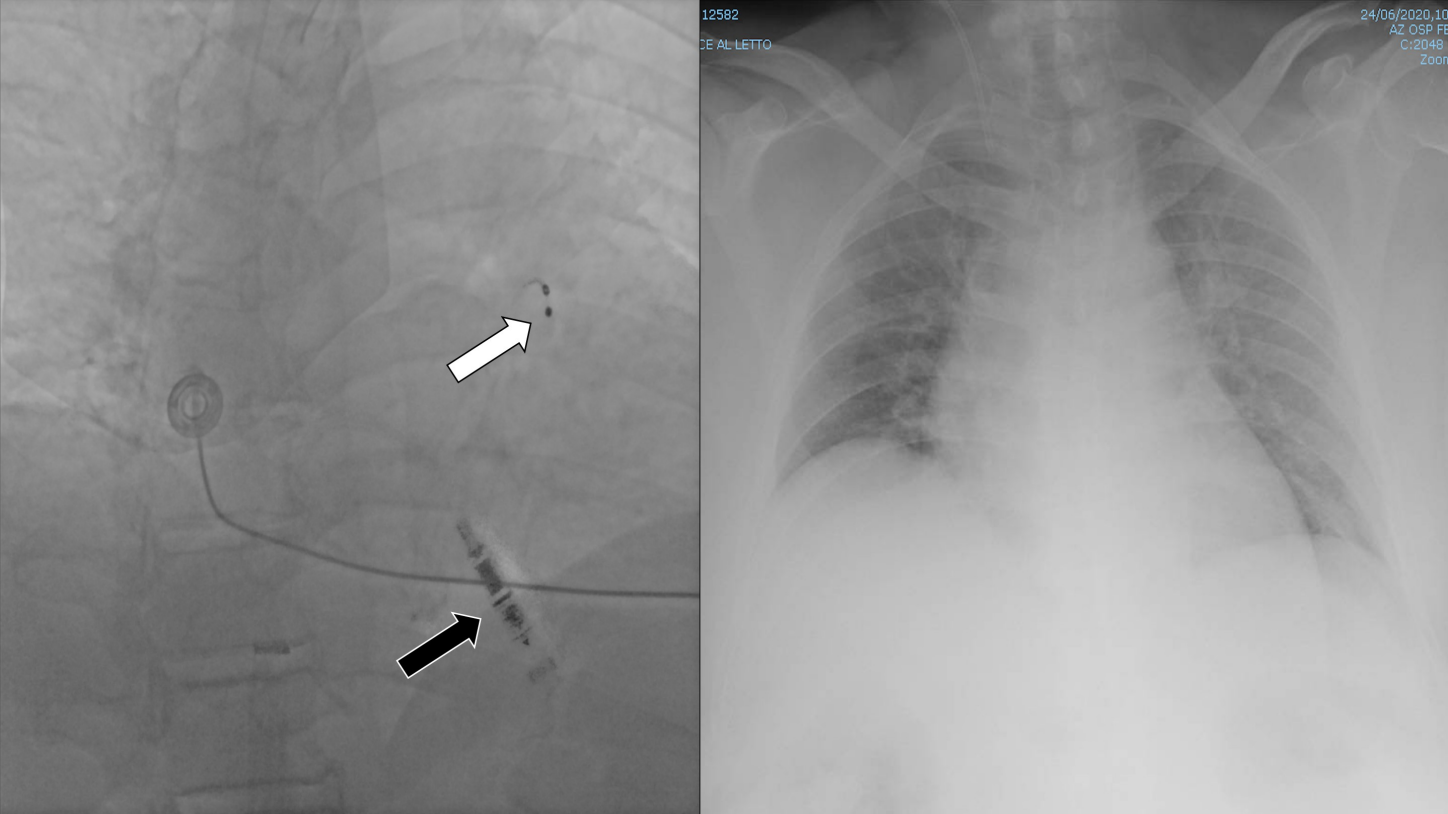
Intraoperative fluoroscopy (left) and postoperative chest X-rays
